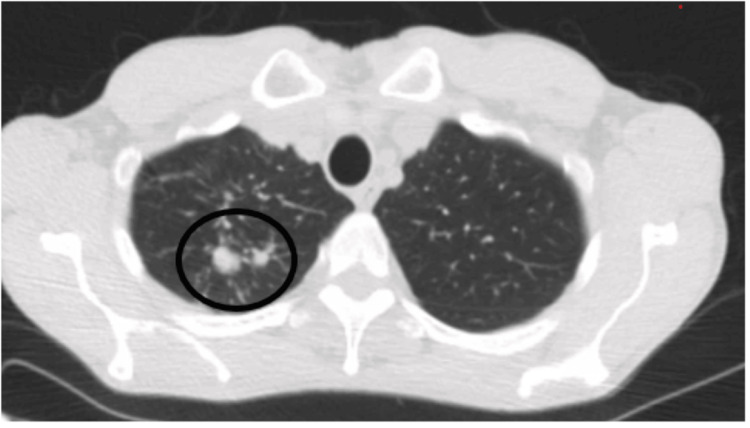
CT scan (axial): right upper 2 spiculated nodules measuring 24 mm and 17.8 mm
